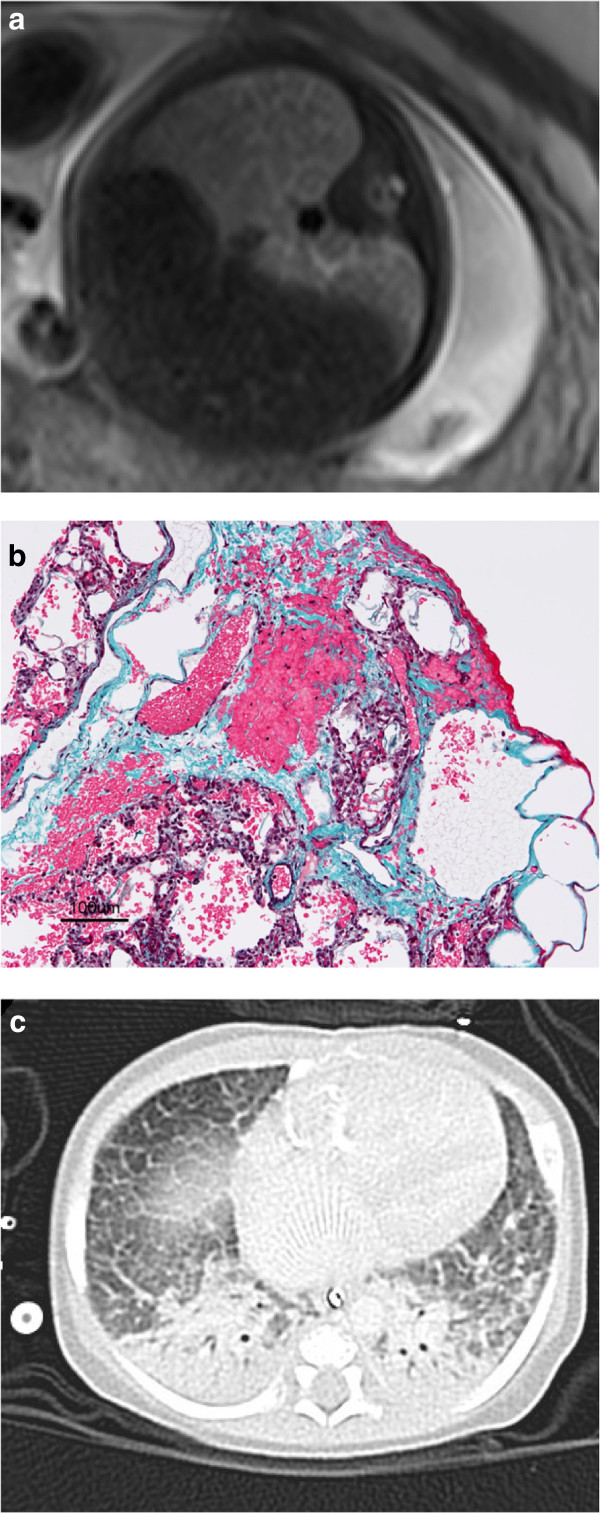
Fetal lung CMR suggestive of pulmonary lymphangectasia confirmed by lung biopsy and postnatal CT in subject with pulmonary venous obstruction. (a) Axial single shot fast spin echo with half Fourier image of the fetal chest demonstrating linear structures extending to the surface of the lung (arrow) suggestive of pulmonary lymphangectasia. The diagnosis was confirmed by (b) lung biopsy showing dilated lymphatics (arrow) and (c) postnatal high resolution CT showing thickening of the interlobular septae (arrow).
